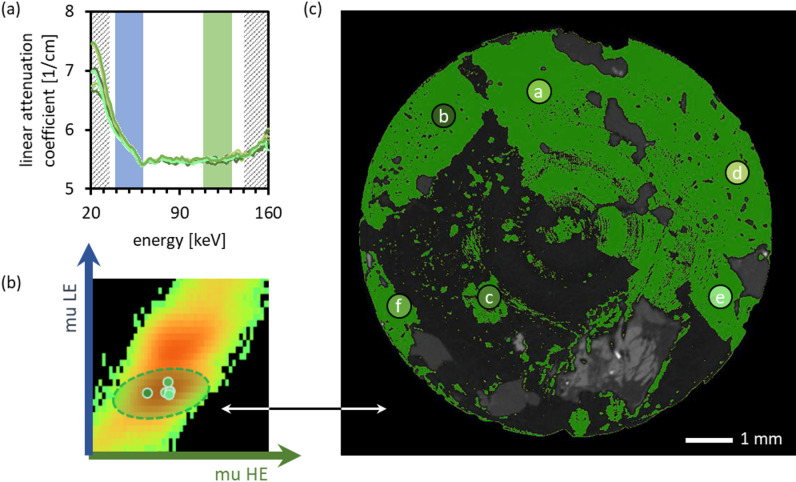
Example of segmentation of phase#1: (a) energy-dependent linear attenuation coefficient of the areas #a to #f selected on (c), the sp-CT image at 50 keV. (b) Zoom on the multi-energy histogram at the location indicated in Fig. 2c. The six areas (#a to #f) are indicated by the six circular markers. The cluster comprising these areas covers phase #1, segmented in green on (c).
